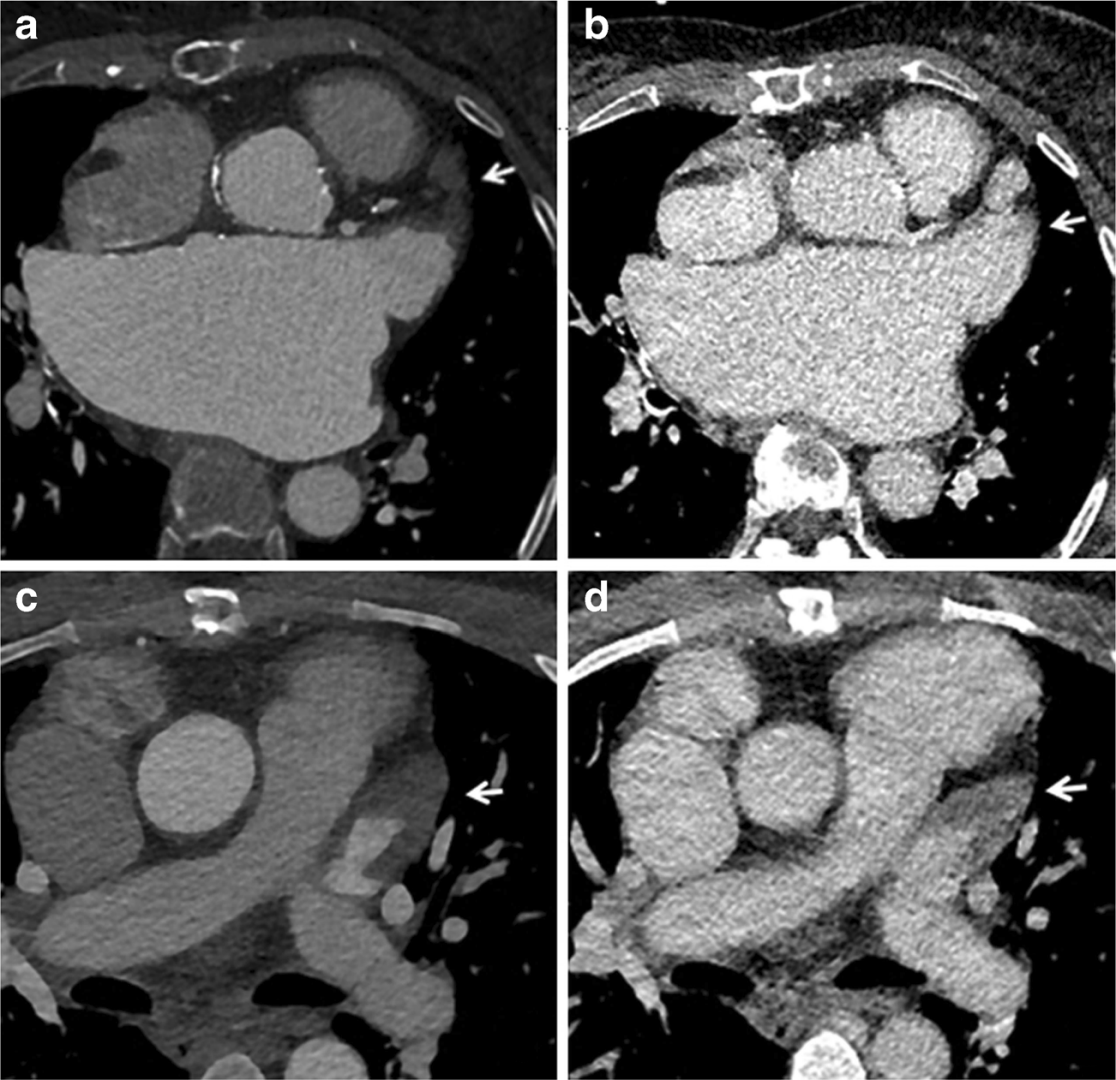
Advantage of a delayed acquisition: differentiating thrombus from flow artefacts. One of the advantages of the delayed scan acquisition is the ability to better depict thrombus and differentiate thrombus from flow artefacts, since patients with a prosthetic heart valve (PHV) often have arrhythmias. In the first patient (A-B), the arterial CT angiography (CTA) acquisition (A) depicts a filling defect in the left atrial appendage (arrow). The delayed scan (B) shows that it involved a flow artefact with good filling of the left atrial appendage in this phase (arrows). Thrombus could thus be excluded. In the second patient (C-D), the arterial CTA acquisition (C) also depicts a filling defect in the left atrial appendage (arrow). However, in this case, the delayed scan (D) confirms the presence of a thrombus (arrow)
